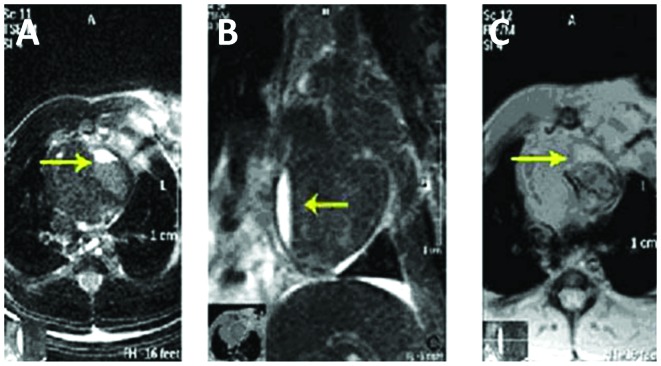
Magnetic resonance imaging of the infarcted region 2 weeks after coronary artery clamping. In the (A) transverse T2* image and (B) oblique sagittal T2* image, high signal intensity was observed in the area of the infarct. (C) In the transverse T2* image, the boundary between the ischemic region and the surrounding tissue was unclear.
